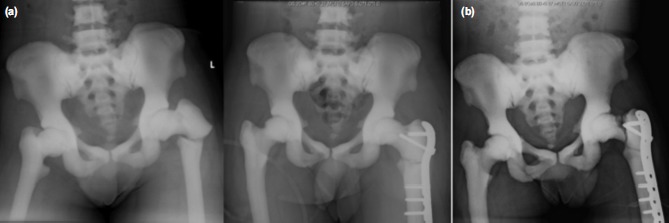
(a) Pre and post-operative radiographs following the fall in 2011 and (b) Left neck of femur fracture sustained in 2014.
